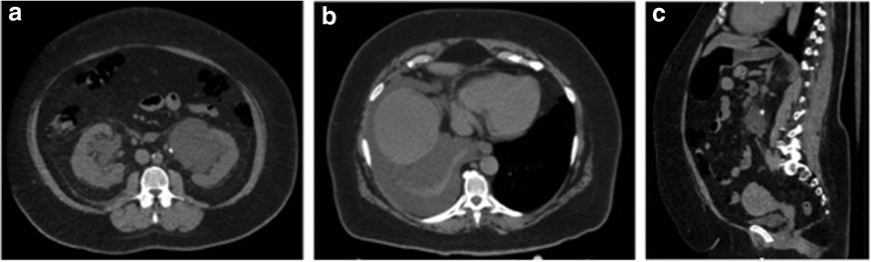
CT imaging performed in the emergency department. (a) Axial view demonstrating presence of left peripelvic cyst. (b) Axial view demonstrating large right-sided pleural effusion. (c) Sagittal view demonstrating obstructing left-sided ureteral calculus.
